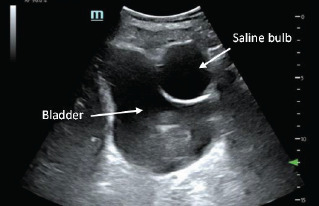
Point-of-care ultrasound image of saline reservoir in pelvic sagittal view.
